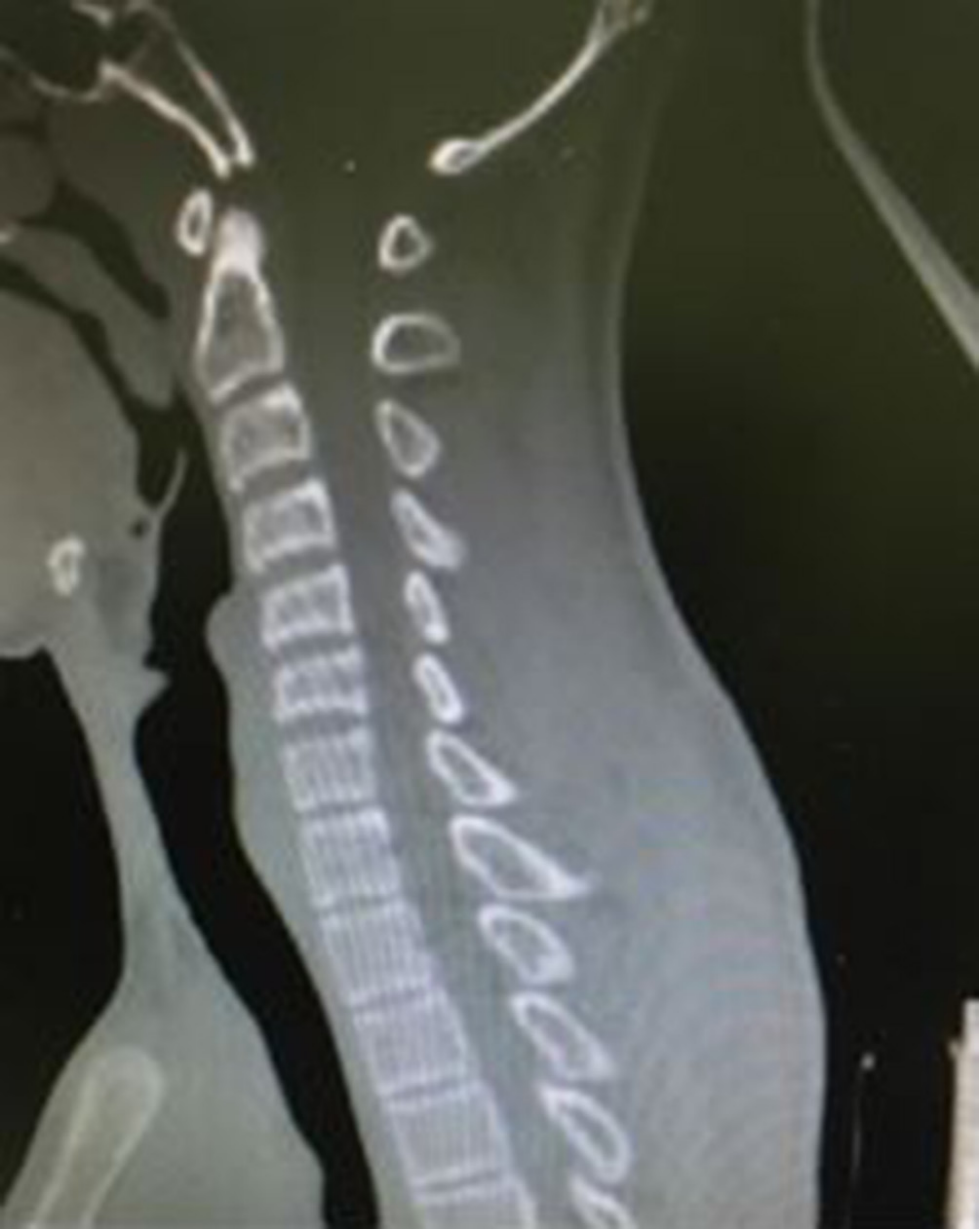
CT cervical spine sagittal reformatted image reveal straightening of cervical
